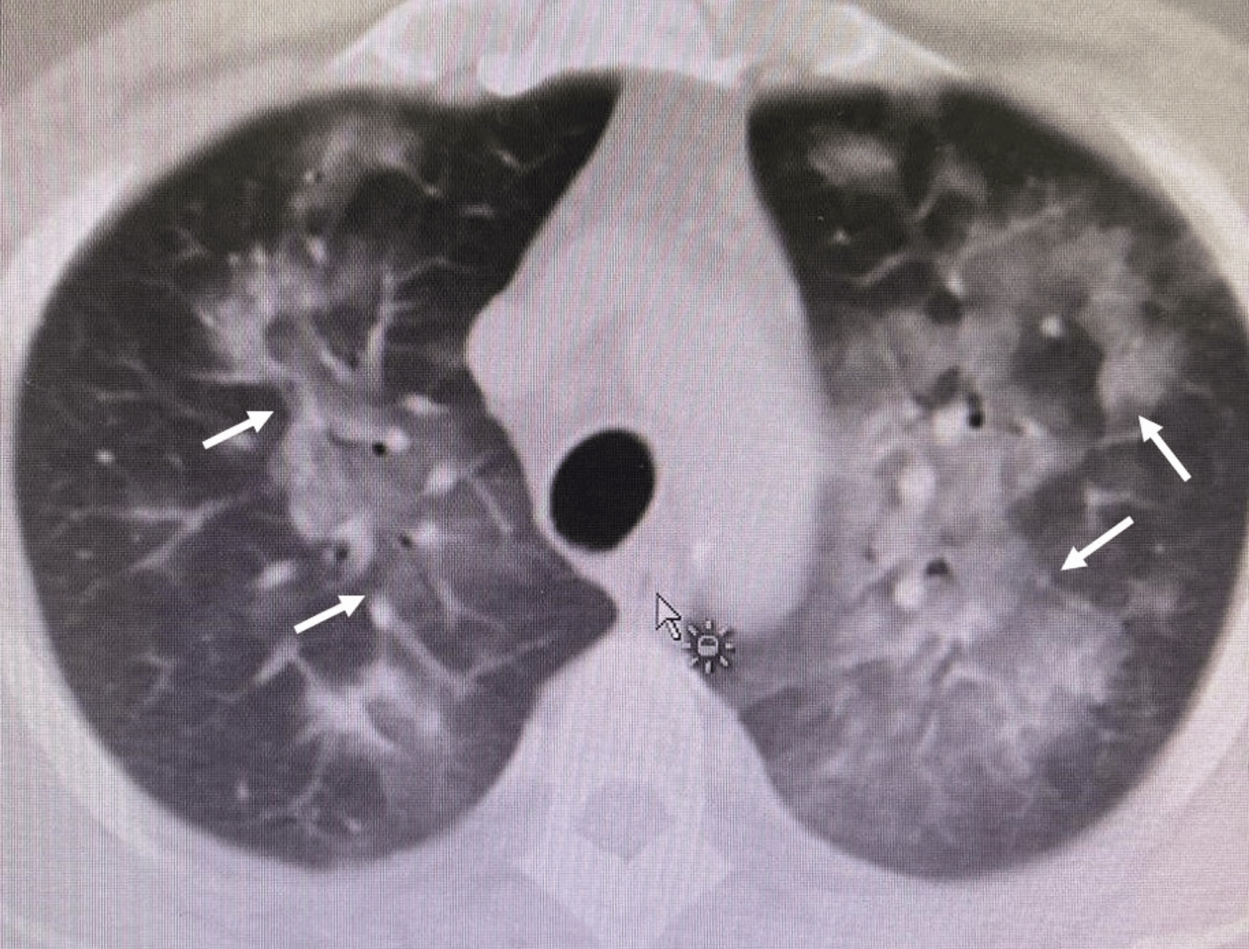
Computed tomography (CT) of the chest showing bilateral lung infection with acute respiratory distress syndrome (ARDS) (arrows).
